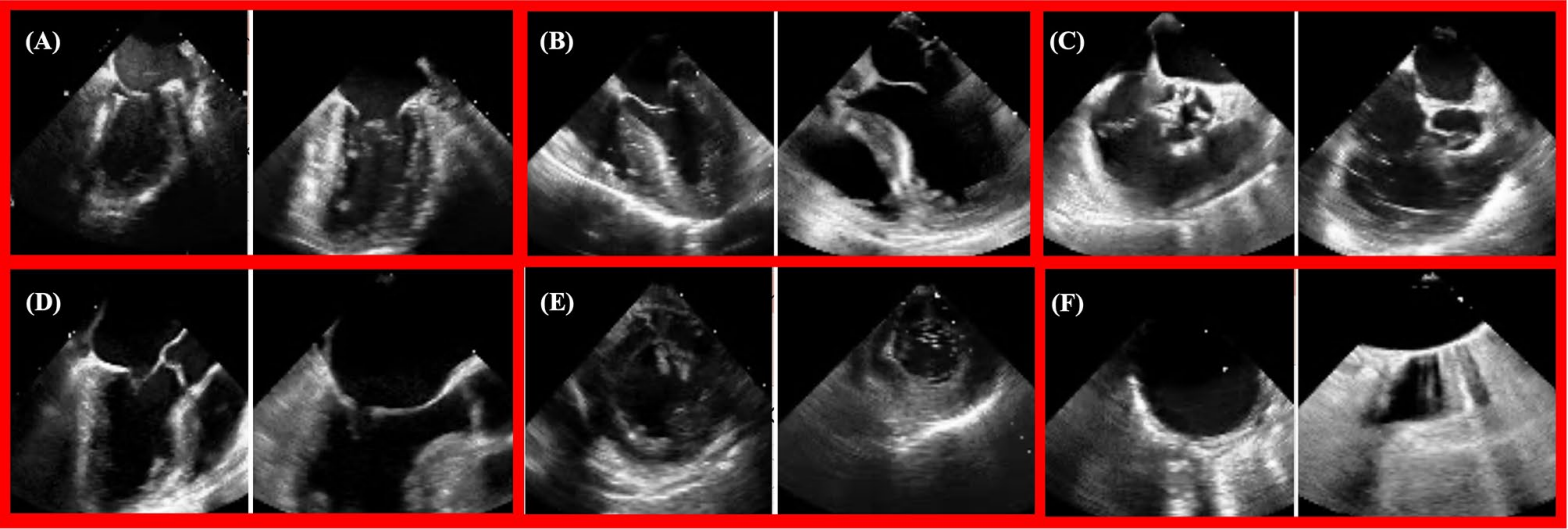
Representation of variation contained within classified views. Six of the eight TEE view class labels represent pooled or generalized categories, reflecting the high degree of anatomical and visual overlap that occurs among the twenty-eight standardized TEE views recommended by the American Society of Echocardiography and the Society of Cardiovascular Anesthesiologists. The images are 2-dimensional still frames sampled from the video data used in model training. (A) The “ME 2-Chamber View” class included ME 2-chamber (left) and ME mitral commissural (right) videos. (B) The “ME 4-Chamber View” class included ME 4-chamber (left) and ME 5-chamber (right) videos. (C) The “ME AV SAX View” class included ME AV SAX (left) and ME RV inflow-outflow (right) videos. (D) The “ME Long Axis View” class included ME long axis (left) and ME AV long axis (right) videos. (E) The “TG LV SAX View” class included short axis videos of the LV at all levels, such as the mid-papillary (left) and the basal (right) levels. (F) The “Aortic View” class included videos of the aorta at all levels, such as the descending thoracic SAX (left) and upper esophageal aortic arch LAX (right) levels. TEE, transesophageal echocardiography; ME, mid-esophageal; AV, aortic valve; SAX, short axis; RV, right ventricular; LV, left ventricular; TG, trans-gastric.
